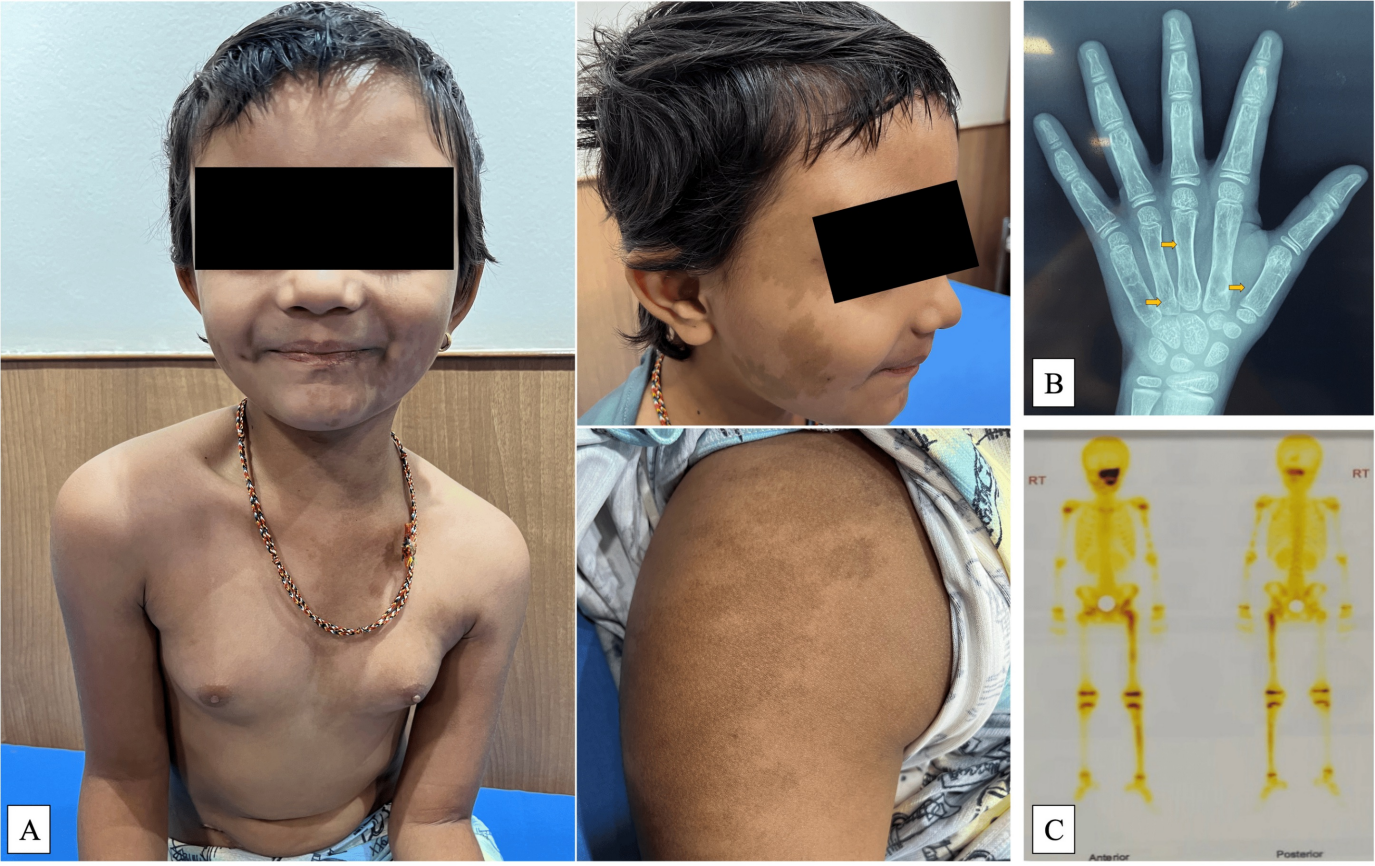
6.6-year-old girl with McCune-Albright syndrome. A. Multiple café-au-lait macules with irregular borders and bilateral breast enlargement, stage 3. B. Polyostotic fibrous dysplasia with left-hand bone age radiograph showing multiple cystic lesions. C. Increased tracer uptake seen on the left side of the skull on bone scan.
